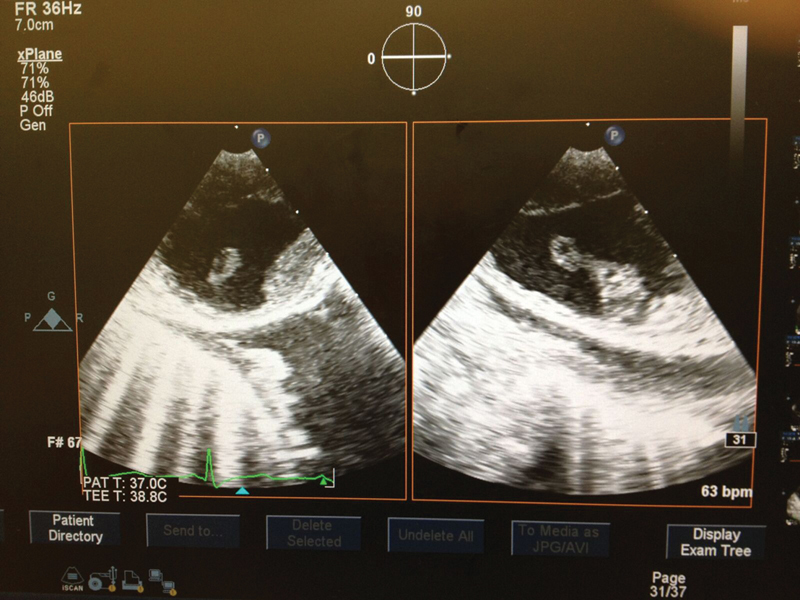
Echocardiography images of the descending thoracic aorta showing intraluminal floating mass.
